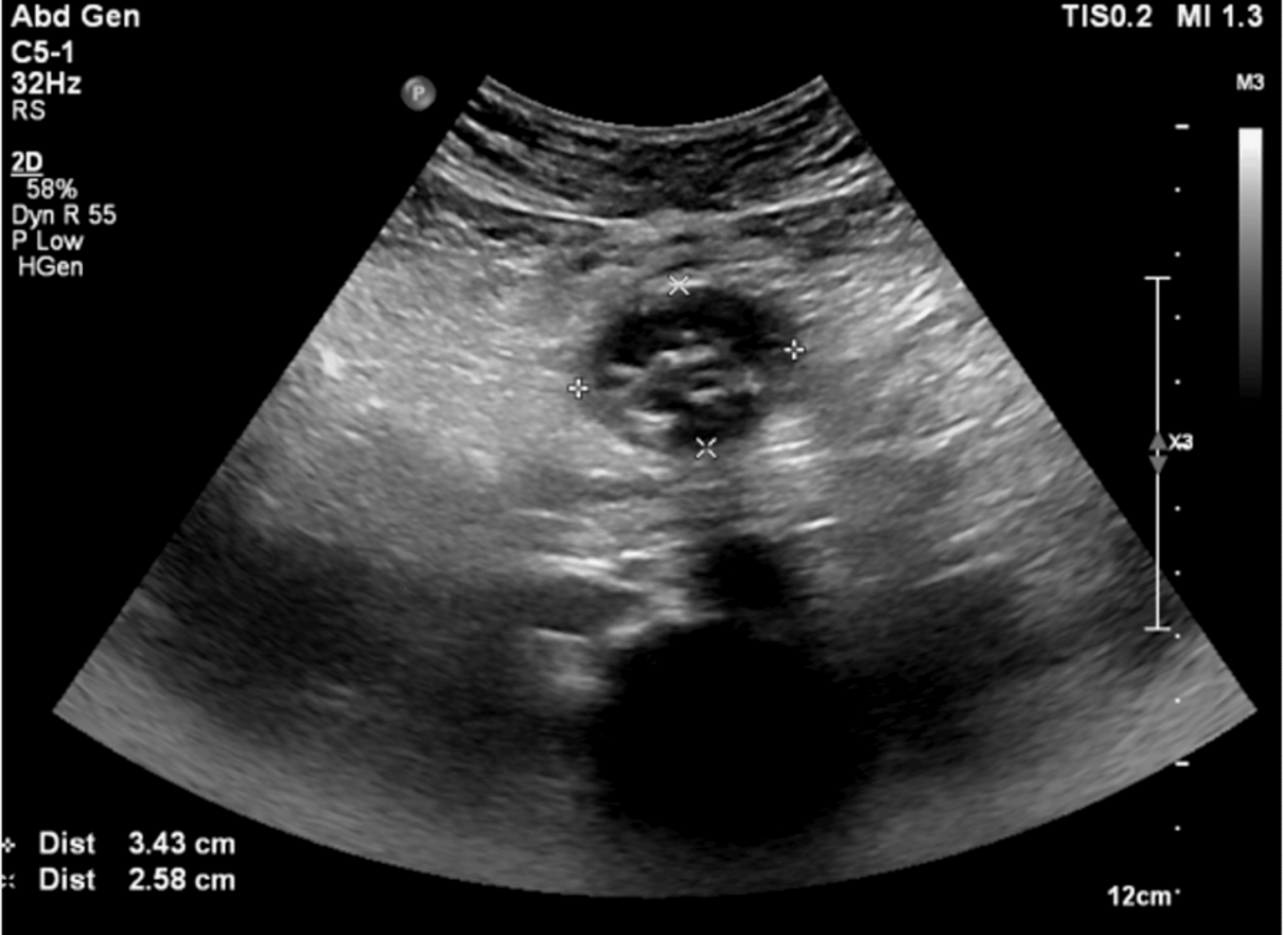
Ultrasonography of the abdomen showing multiple calculi in the gallbladder and a well-defined heteroechoic cystic area with thick walls and a few echogenic foci in the region of the omentum
